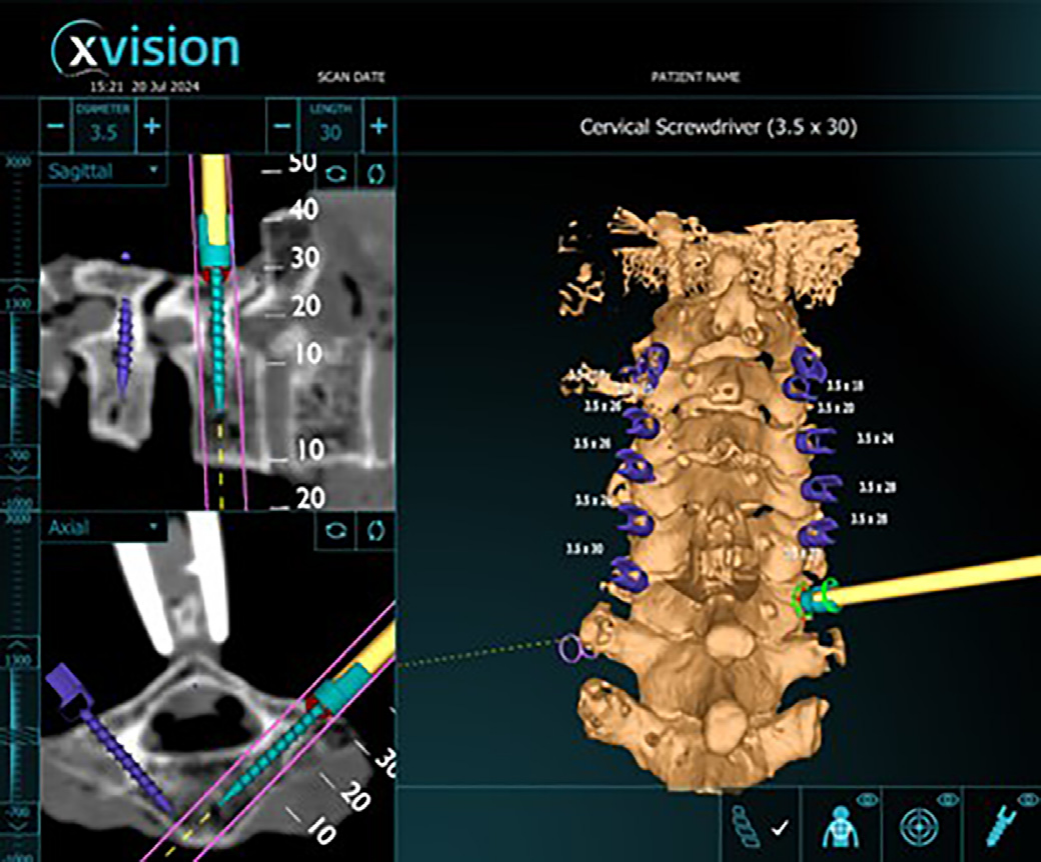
Screen shot showing final planned screw on axial CT scan, sagittal CT scan, and 3D model.
